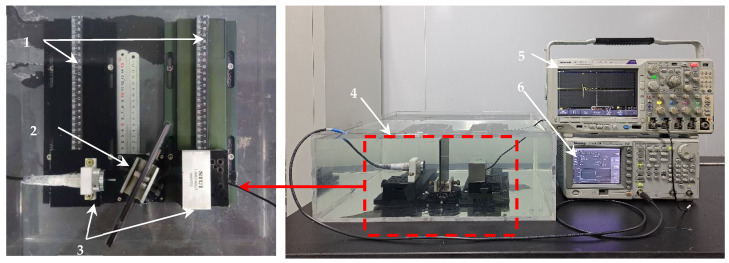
Water immersion ultrasonic transmission wave experimental detection system. 1: Guide; 2: Turntable; 3: Water immersion ultrasonic sensor; 4: Sink; 5: Oscilloscope; 6: Signal generator.
